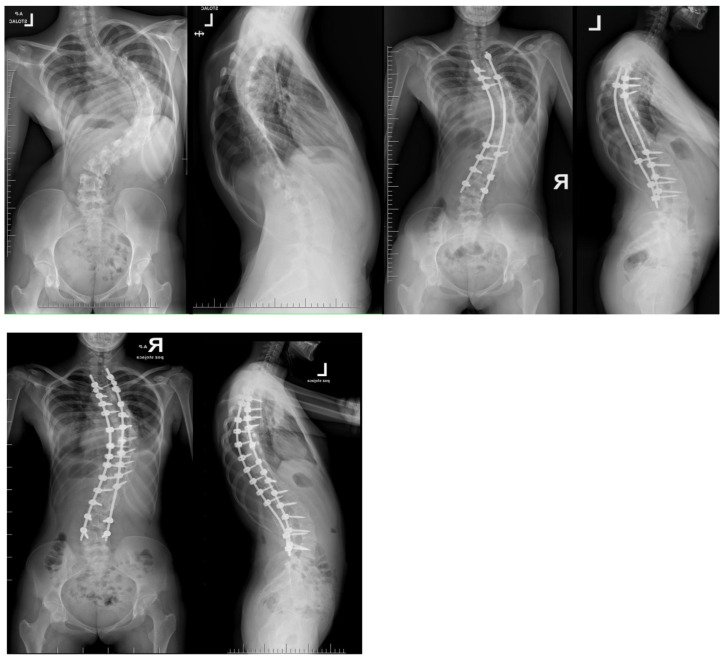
A 17-year-old female patient underwent a series of surgical procedures involving the less invasive temporary internal distraction technique followed by posterior spinal fusion. Radiographs were conducted before the initial stage, after the first stage, and post-operatively during the final follow-up.
